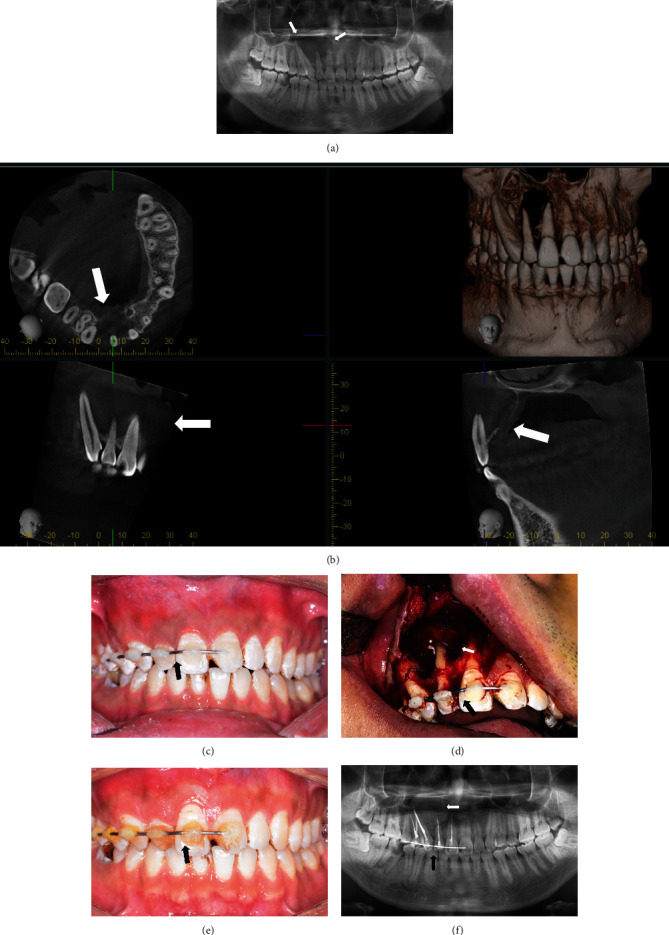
A 24-year-old male presenting with odontogenic keratocyst in the right maxilla involving 4 teeth (case 4). (a) Routine panoramic radiograph revealed a maxillary lesion with well-defined lesion, involving #11, 12, 13, and 14 (white arrow). (b) CBCT examination showed that #11, 12, 13, and 14 lost bone support (white arrow). (c) #15, 14, 13, 12, 11, and 21 were splinted before surgery (black arrow). (d) There is a large bone defect caused by lesion and surgery, and teeth had minimal bone support (white arrow). But no tooth was avulsed or excessive mobile during surgery, and the splint was fixed well on the teeth (black arrow). (e) One month after surgery, the resin of the splint had slight discoloration (black arrow). (f) Teeth were preserved, and bone defect was filled with biomaterial (white arrow).
